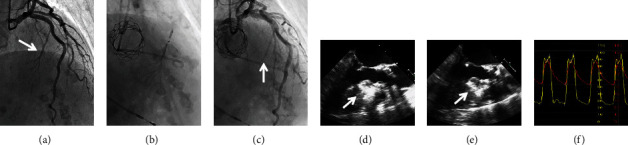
(a) The first septal branch was confirmed beforehand. (b) Tip injection into the septal branch using a 2 mm balloon. (c) Total occlusion of the septal branch was confirmed after alcohol injection. (d) Transesophageal echocardiography confirmed the perfusion area of the septal branch as a bright area by contrast injection. (e) Alcohol was administered into the septal branch. (f) Finally, pressure gradient at the left ventricular outflow tract was decreased.
